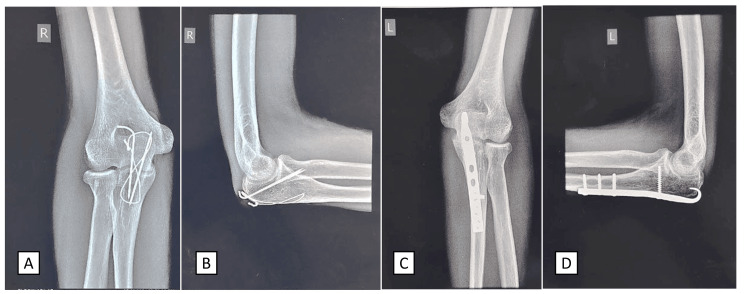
Postoperative radiographs of the left and right elbows taken two years after surgery (A) Anteroposterior X-ray view of the right elbow with tension band wiring in situ; (B) lateral X-ray view of the right elbow with tension band wiring in situ; (C) anteroposterior X-ray view of the left elbow with an anatomical plate in situ; (D) lateral X-ray view of the left elbow with an anatomical plate in situ.
